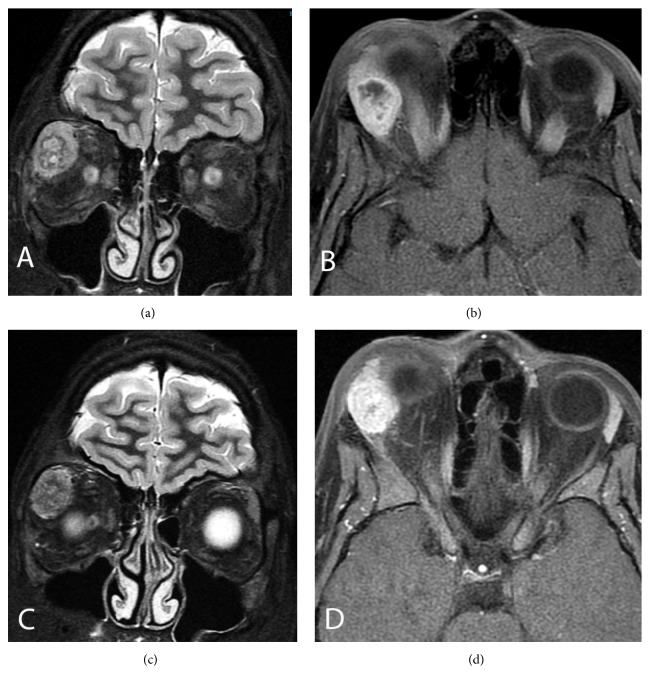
Coronal T2-weighted MR image reveals a heterogeneous oval-shaped right lacrimal gland mass with low and high signals containing solid and cystic components (a). Fat-saturated axial postcontrast MR image obtained at 20 months before the admission shows heterogeneous intense enhanced mass due to unenhancing cystic component (b). Coronal T2-weighted MR image shows a heterogeneous oval-shaped predominantly solid mass with low and high signals without a clear cystic component (c). Fat-saturated axial postcontrast MR image on admission shows almost homogeneous intense enhancement (d).
